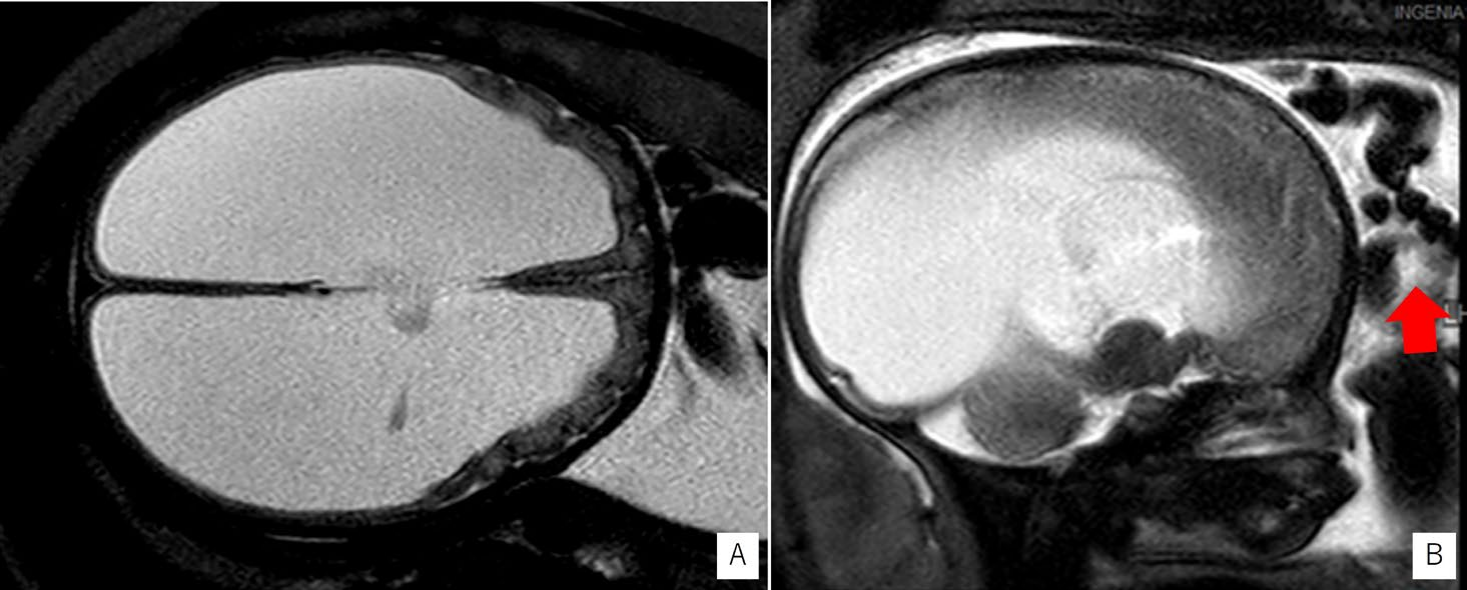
Fetal MRI images at 34 weeks of gestation. (A) Axial T2‐weighted image showing marked enlargement of the lateral ventricles. (B) Sagittal T2‐weighted image showing adduction and flexion of the left thumb (red arrow). T1‐weighted images were not acquired in this case.
